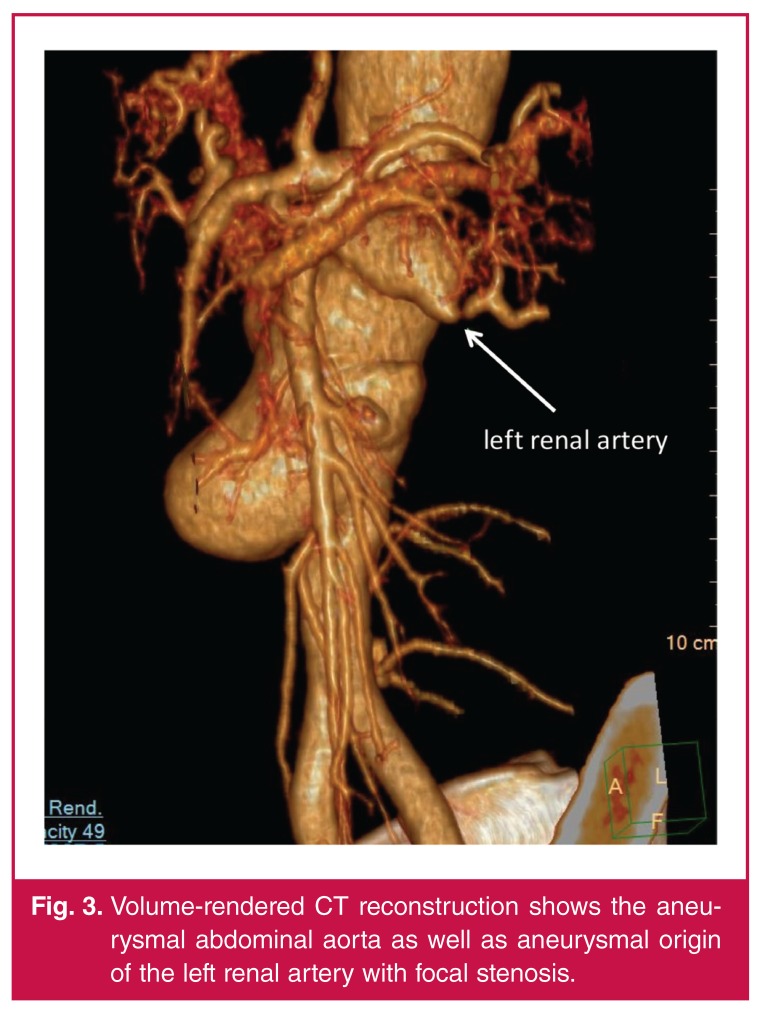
Volume-rendered CT reconstruction shows the aneurysmal abdominal aorta as well as aneurysmal origin of the left renal artery with focal stenosis.
